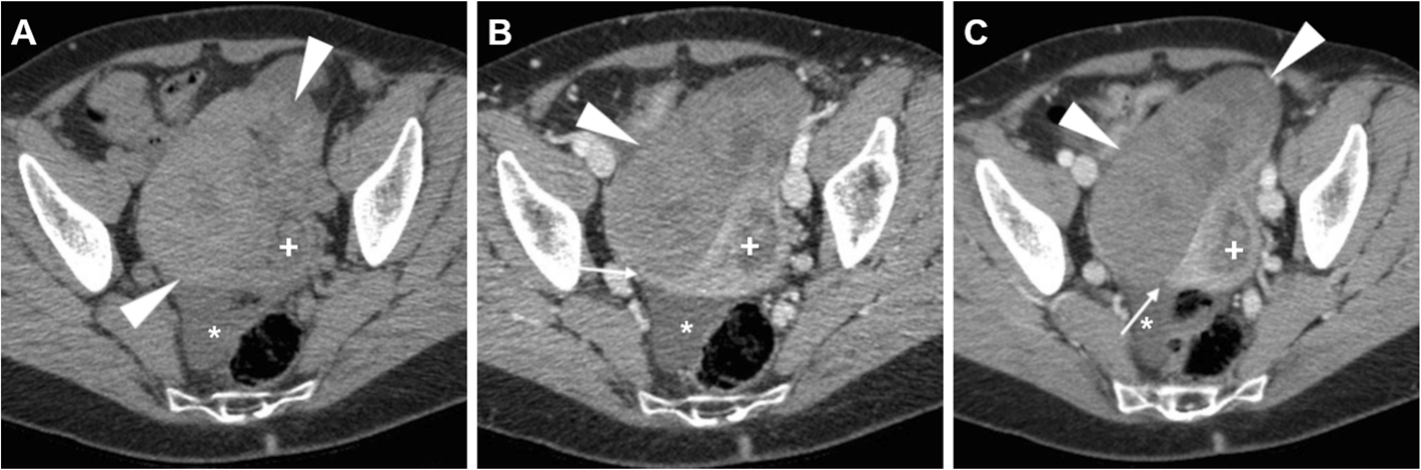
Surgically proven torsed uterine fibroma in a 64-year-old woman. Precontrast (a), arterial (b) and venous (c) phase CT images show a large, ovoid-shaped heterogeneous, mildly hyperattenuating and poorly enhancing mass (arrowheads) that displaces the uterus (plus sign) posterolaterally. Note the acute angle (thin arrow) indicating subserosal origin of the mass, and minimal effusion (asterisk) in the peritoneal cul-de-sac
